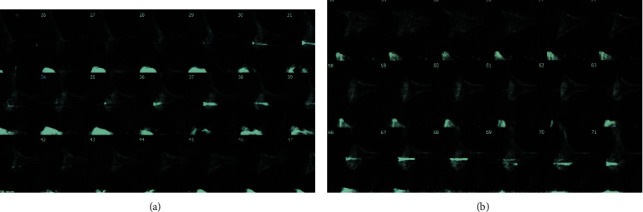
Sagittal view of CBCT images in case no. 2: (a, b) grafted areas 6 months postoperatively.
